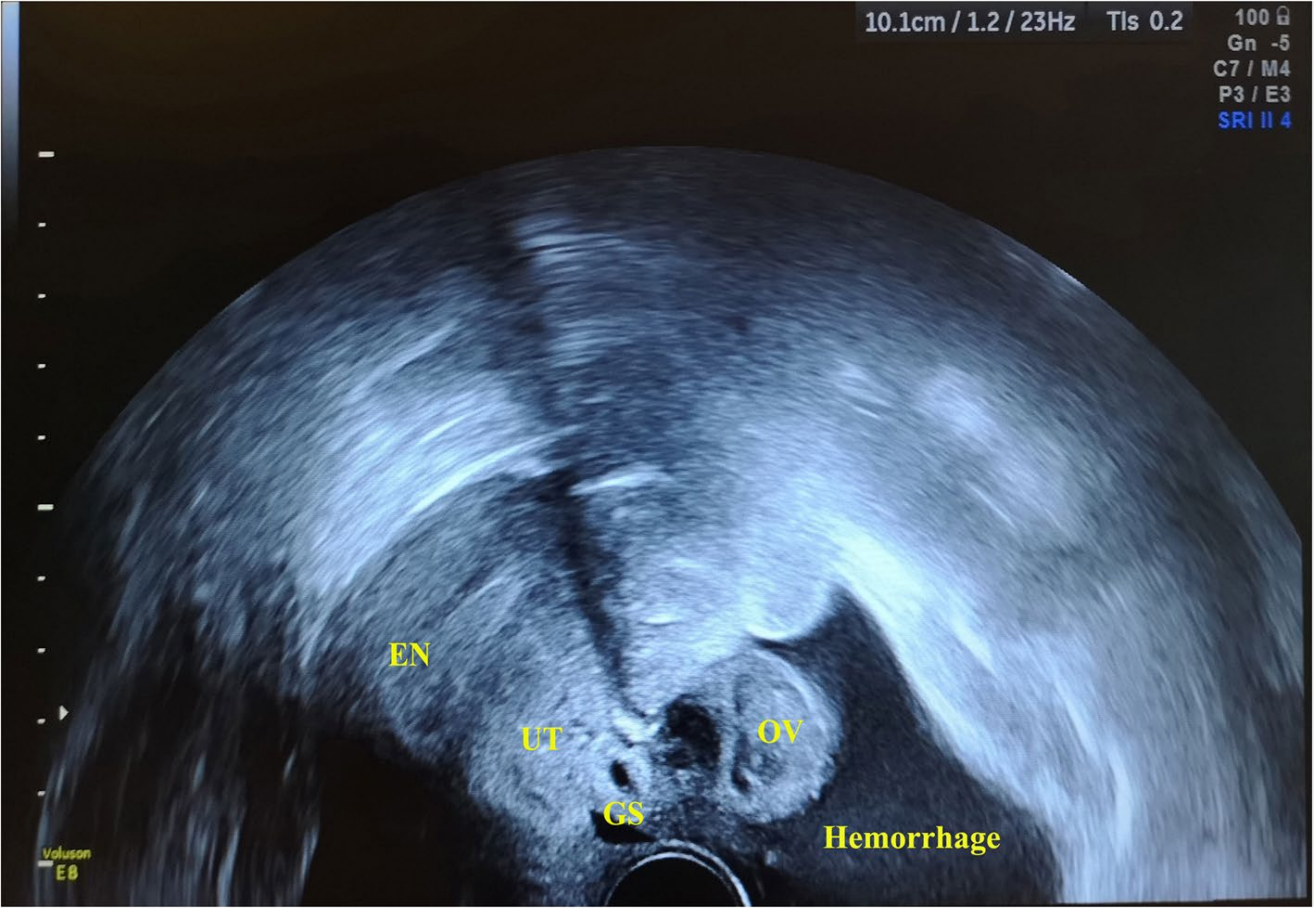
Transvaginal ultrasonography showed the intra-abdominal hemorrhage (96*57 mm) in the pelvic cavity and enlarged ovaries with multiple corpus luteums. It identified a 13 mm × 11 mm-sized GS-like echo near the posterior surface of uterus. EN: endometrium; UT: uterus; GS: gestational sac; OV: ovary
